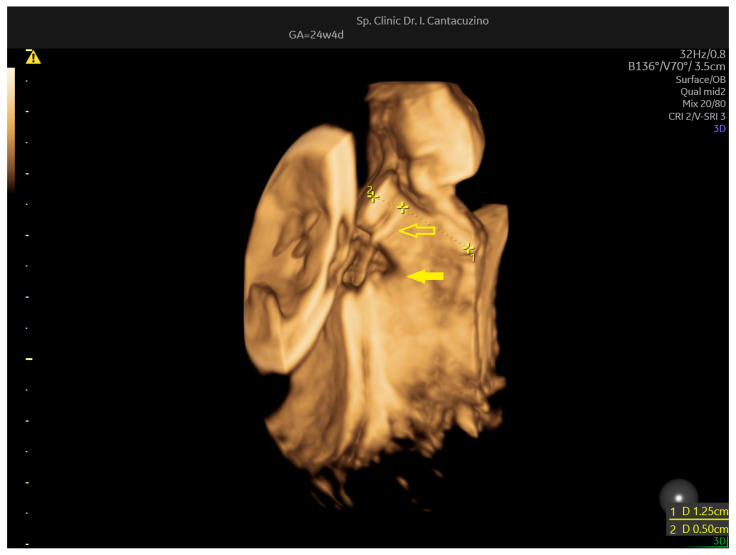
Patient no. 3. Transvaginal ultrasound at 24 weeks, triggered by asymptomatic contractility. Parasagittal section reconstruction revealing cerclage thread. Empty arrow points to cerclage thread. Internal distance was 5 mm. External distance was 12.5 mm. Solid arrow points to tendency for funneling below the thread.
